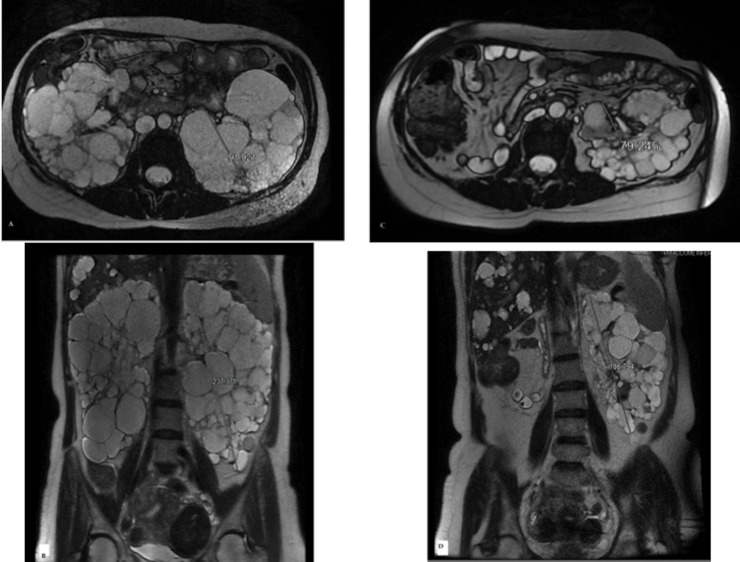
Magnetic resonance imaging of a 52 year-old woman, with end-stage renal disease due to autosomal dominant polycystic kidney disease. Pretransplant Axial T2 weighted (A) and Coronal T2 weighted images (B) demonstrated a massive enlargement of both kidneys with the lower pole in the iliac fossa. The patient underwent a kidney transplantation with simultaneous right nephrectomy, and 1-year Axial T2 weighted (C) and Coronal T2 weighted images (D) demonstrated a significant reduction of kidney volume.
